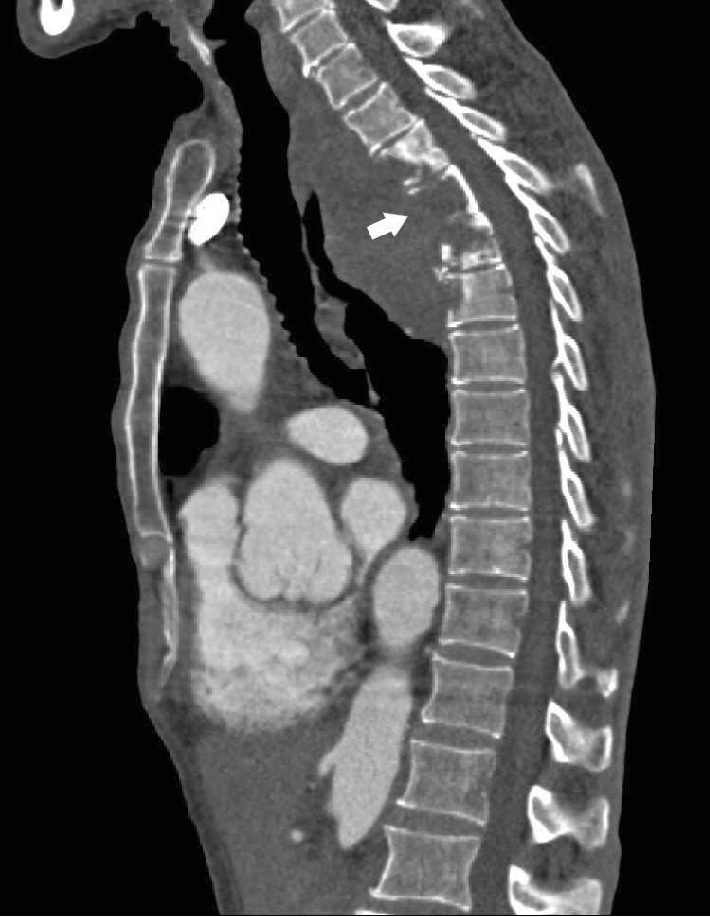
Sagittal section of a chest CT angiography showing the lysis of the vertebral bodies of D2, D3, D4, and D5 without displacement of the posterior wall.
